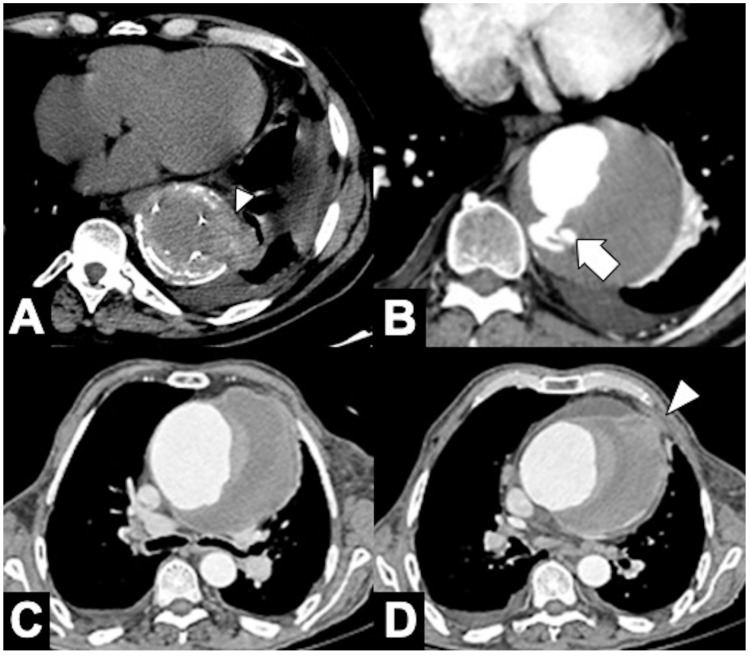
CTA findings in impending rupture of the thoracic aorta aneurysms. (A) Missing calcium sign (arrowhead). (B) Thrombus fissuration (arrow). (C) Hyperattenuating crescent sign, periaortic stranding sign, and contour irregularity of the aneurysm. (D) Periaortic fat stranding blurs a focal area of the mediastinum and extends to the chest wall representing an inflammatory process (arrowheads) [111].
